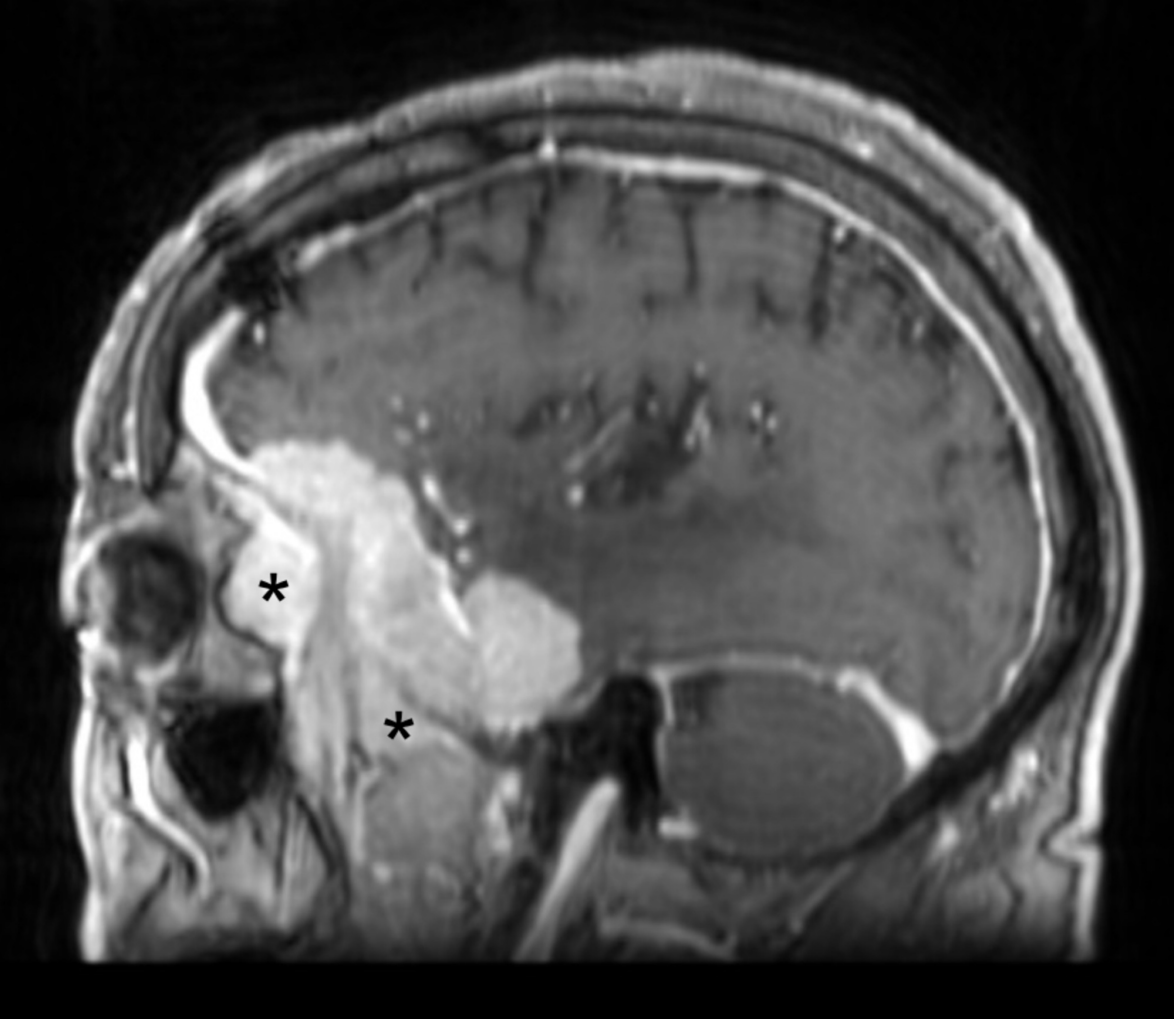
Preoperative sagittal MRI demonstrating tumor involvement of the lateral orbit and temporalis muscle * = Tumor invasion of the lateral orbit and temporalis muscle MRI: magnetic resonance imaging
